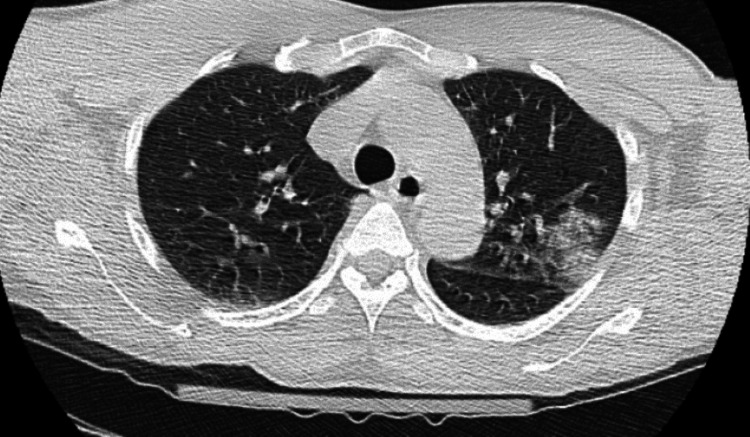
CT chest demonstrating left upper lobe pneumonia
